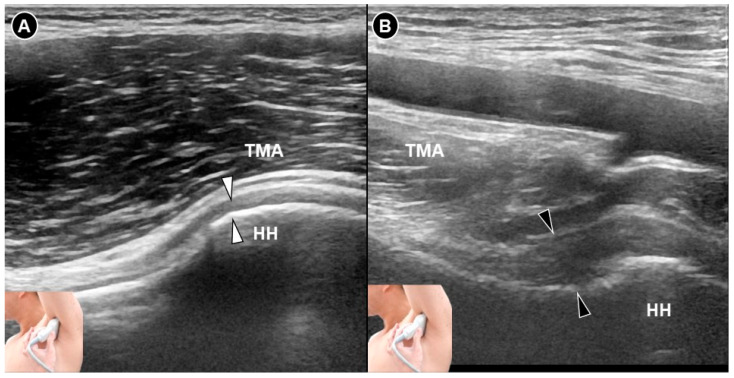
Ultrasound imaging of the inferior glenohumeral joint *(white arrowheads)* in a normal shoulder (A). In contrast, imaging of the inferior glenohumeral ligament *(black arrowheads)* in a shoulder with adhesive capsulitis reveals thickening of the inferior glenohumeral capsule (B). HH, humeral head; TMA, teres major.
